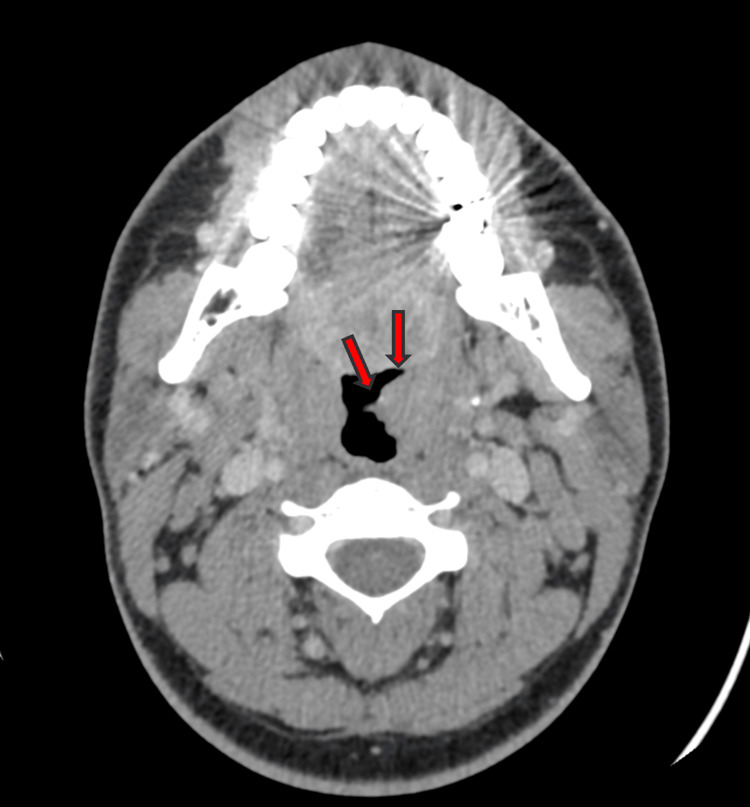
Axial CT image showing left palatine tonsillar hypertrophy (arrows)
